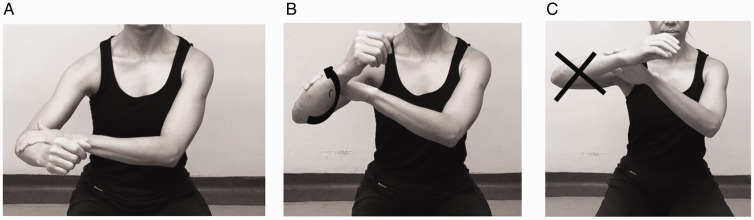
Posterior deltoid and teres minor. Isometric abduction and external rotation in the scapular plane. A, Initial position: affected arm is kept at 30° of scapular plane abduction and the forearm is pressed against the opposite hand outward. B, Progression: affected arm is externally rotated while resisting against the opposite hand and keeping the elbow inward. C, The hornblower sign, commonly seen in patients with CTA should be avoided by focusing on external rotation and moving the elbow inward.
